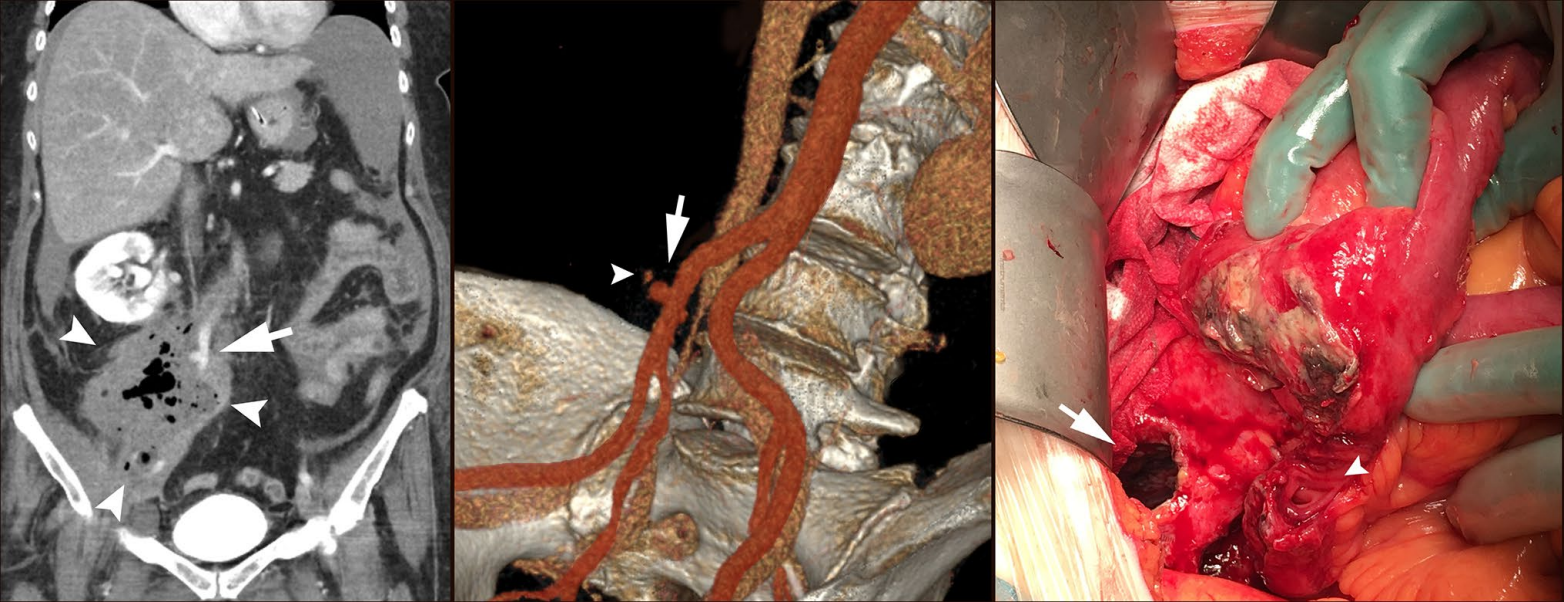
Left: coronal abdominal computed tomography with right sided retroperitoneal abscess (small arrows) and pseudoaneurysm (large arrow). Middle: 3-D volume computed tomography reconstruction of abdominal vessels, pseudoaneurysm in right common iliac artery (large arrow) and minimal extravasation (small arrow). Right: per-operative laparotomy, opening of the abscess (large arrow) after small bowel adherent to the abscess has been retracted with one of the fistula in the ileum is presenting with visible mucosa (small arrow)
